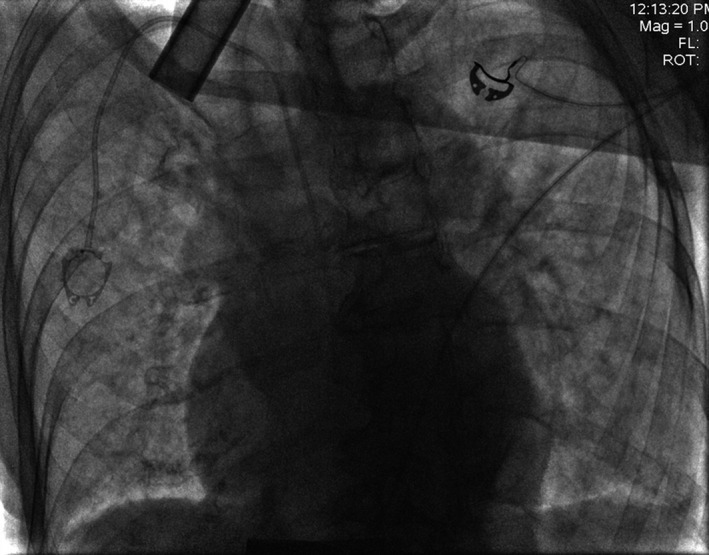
Chest radiography after initial right Port‐A‐Cath placement.
